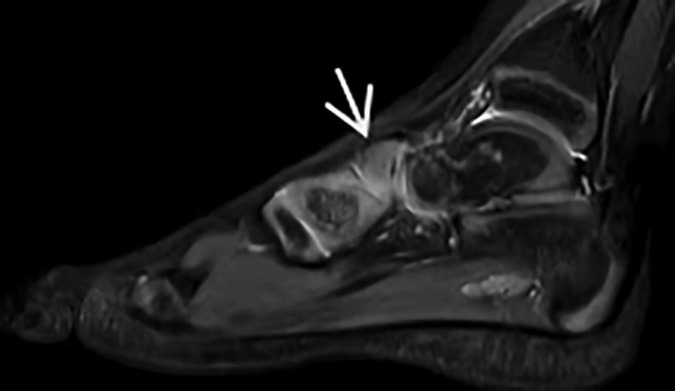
Dorsal talonavicular subluxation in the sagittal plane of MRI (arrow).
